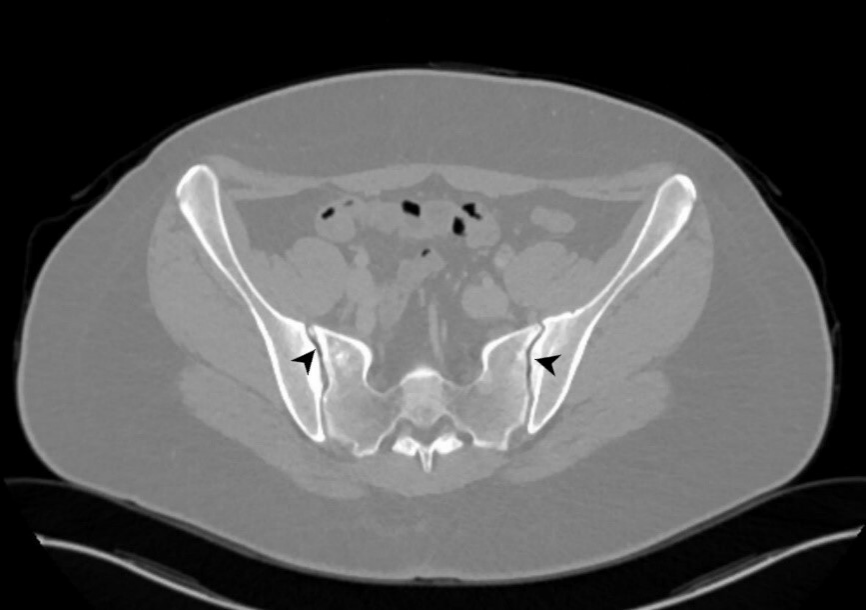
Bilateral air densities corresponding to VP in SIJs (arrows) on axial CT in the bone window.
